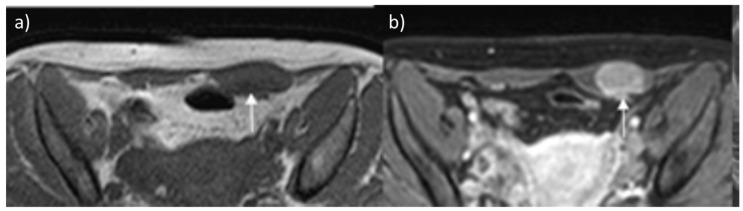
Desmoid tumor on MR. Axial T2 (a) and T1-weighted (b) contrast-enhanced scan show the typical appearance of a long-standing desmoid tumor (arrow), which is almost indistinguishable from surrounding muscles. After contrast, the mass shows brisk and homogeneous enhancement.
